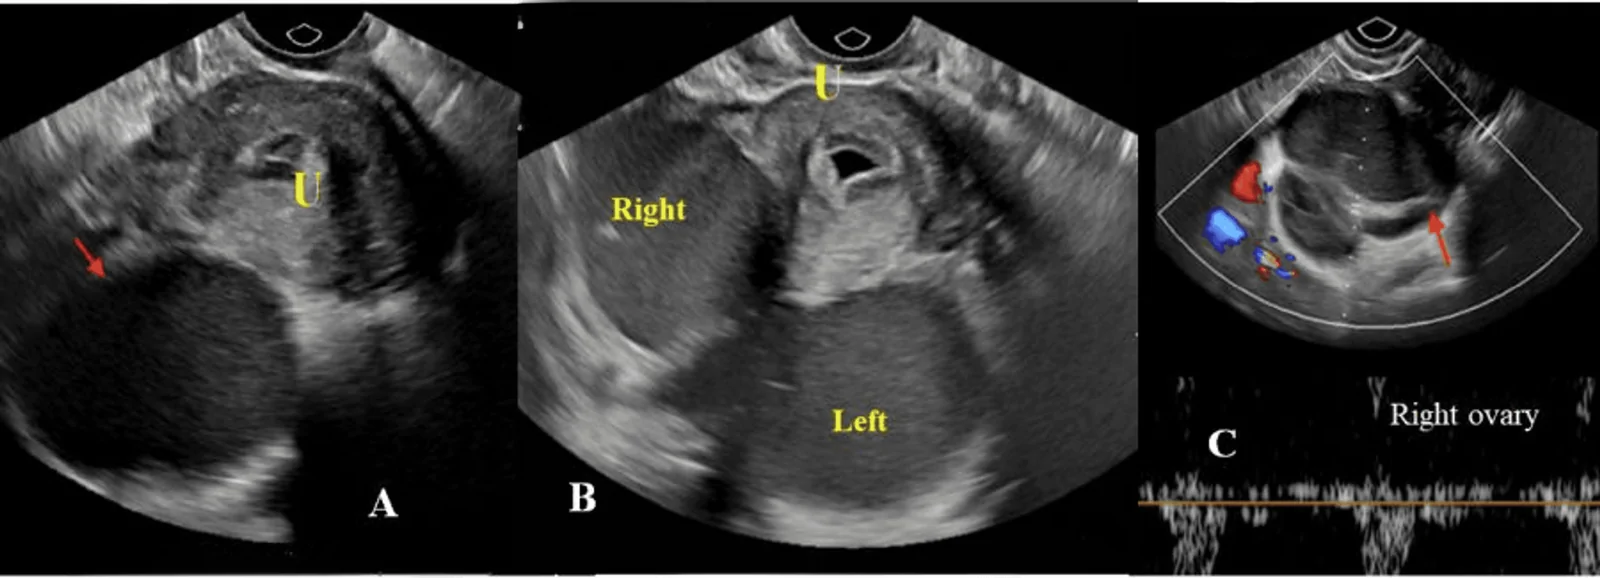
TVUS demonstrating bilateral complex ovarian lesions "A" shows a longitudinal scan showing the uterus ("U") and an ovarian cyst (red arrow) in the cul-de-sac, and "B" shows a transverse scan showing the right ovary superior to the left ovary. The right ovary measures 7.6 × 6.0 × 5.6 cm, and the left ovary measures 5.2 × 3.7 × 2.4 cm. Both ovaries demonstrate homogeneous echogenicity with low-level echoes, interpreted as either hemorrhagic cysts or endometriomas. "C" shows a color Doppler image of the right ovary showing venous flow within the capsule and no vascular flow within the ovary itself. The red and blue colors represent blood flow within the vascular structures, and the red arrow indicates one of the cystic cavities. TVUS: transvaginal ultrasound
